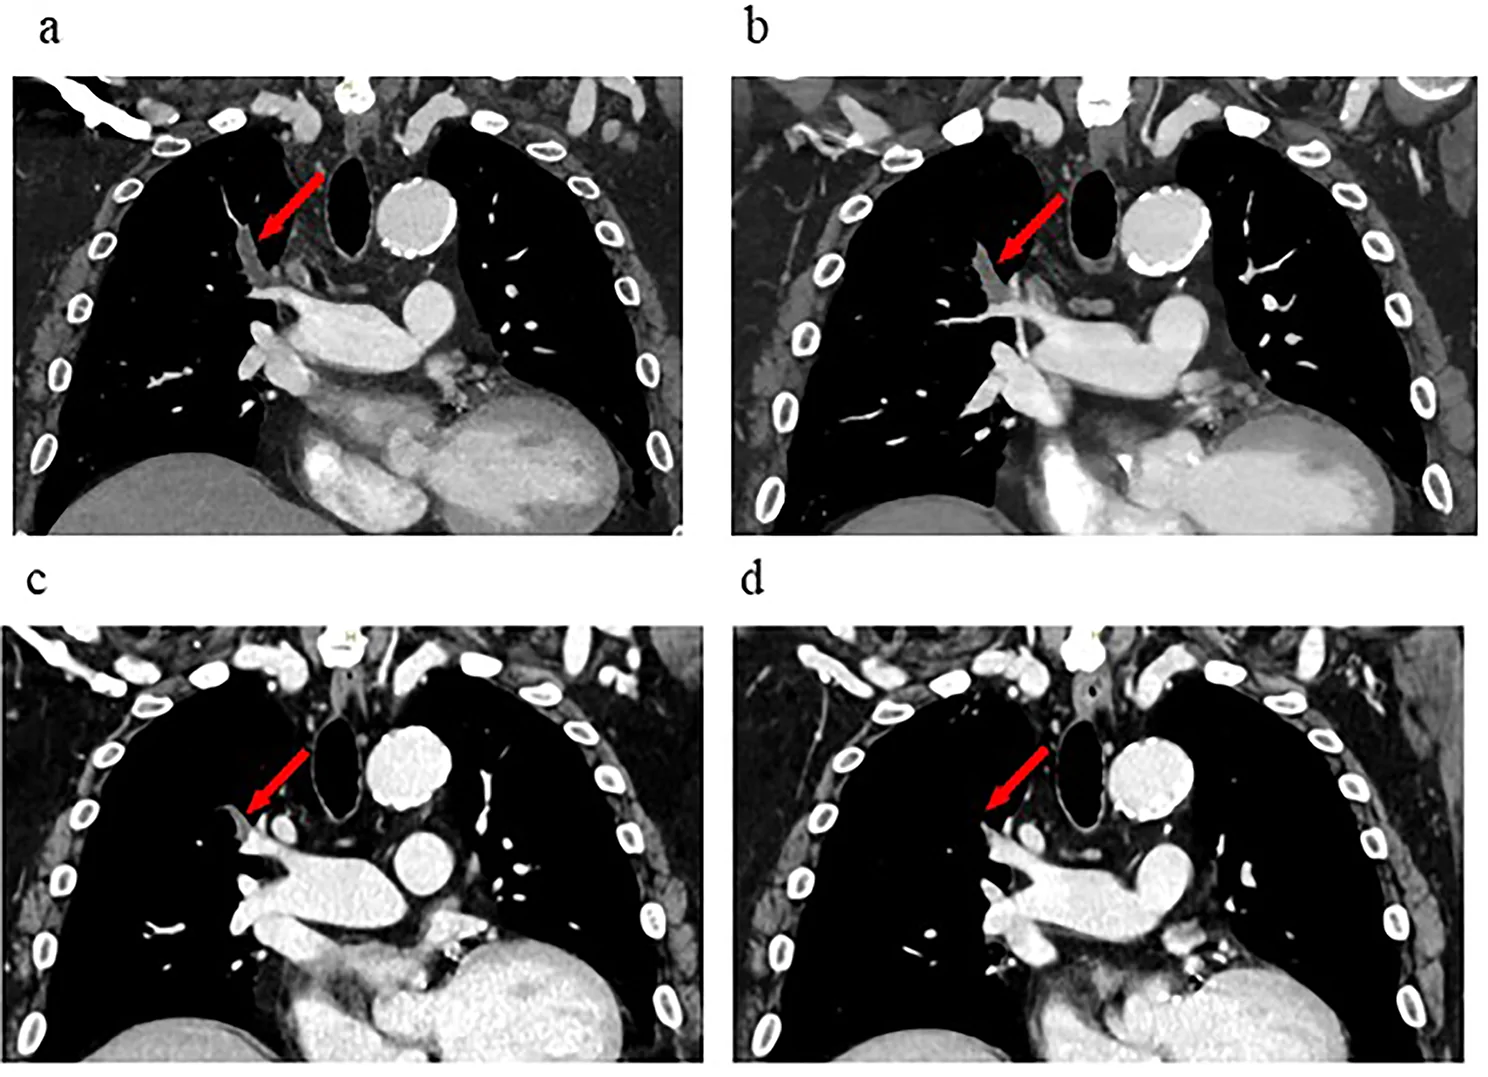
Abdominal CT after starting warfarin treatment. (a) Days 5, (b) 19, (c) 43, and (d) 133. Abdominal CT showed a reduction of right pulmonary artery embolism. CT: computed tomography
